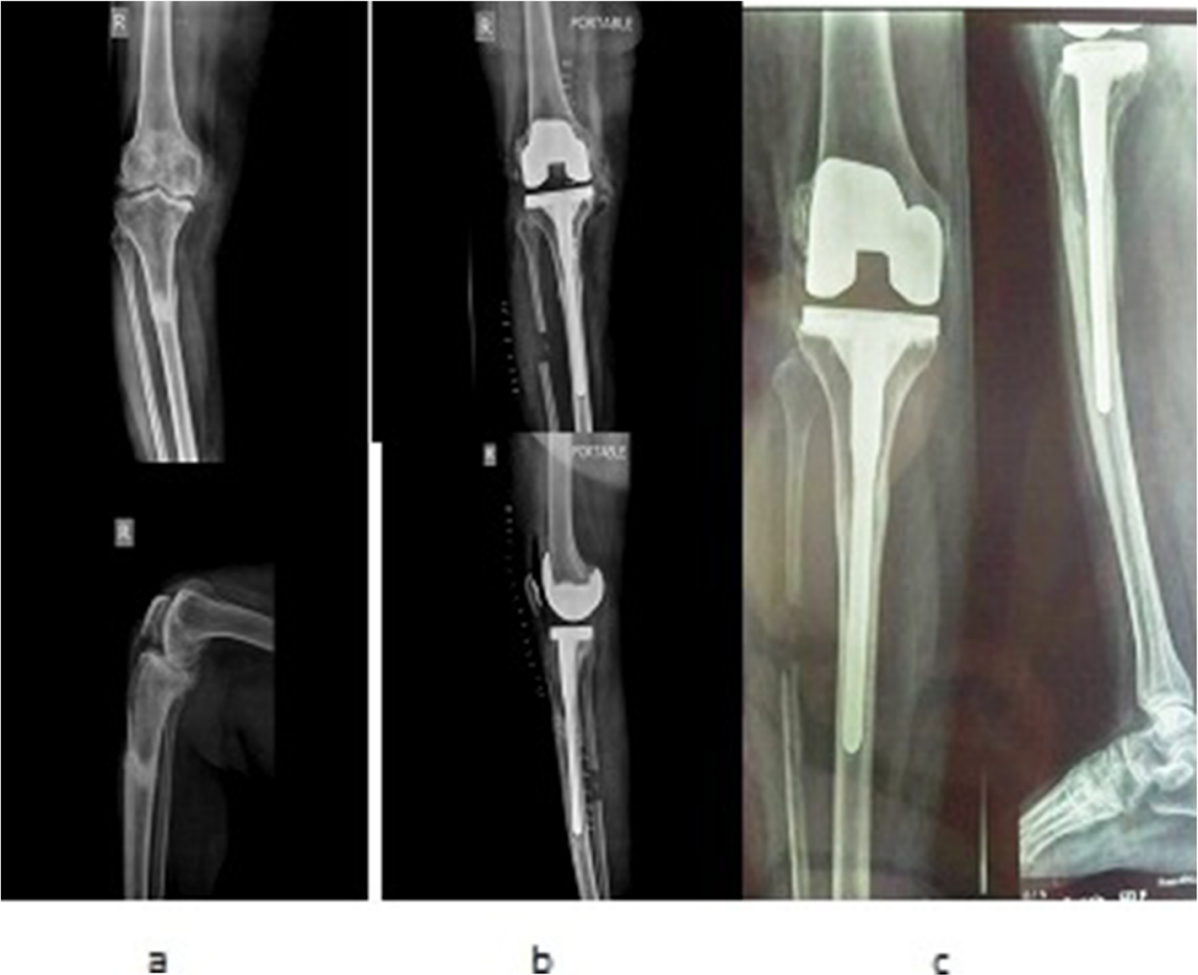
Right Knee X-rays. a) Preoperative anteroposterior and lateral view showing features suggestive of osteoarthritis, with deformity with stress fracture proximal tibia; b) Postoperative X-ray anteroposterior and lateral view showing correction of deformity with modular stemmed knee prosthesis with proximal fibular resection; c) Recent follow-up anteroposterior and lateral view showing healed stress fracture with correction of deformity with modular stemmed knee prosthesis in situ with proximal fibular resection
